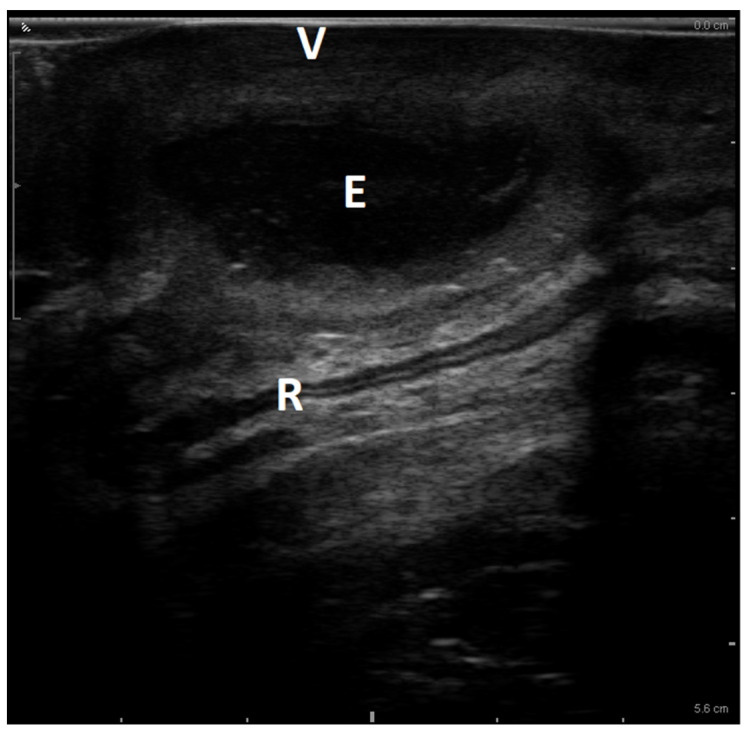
Transvaginal midsagittal sonographic view of the infected rectovaginal endometriosis, using an endocavitary linear probe. Ultrasound evaluation showed an inhomogeneous iso-hypoechoic 3–4 cm lesion involving the rectovaginal septum, but the integrity of the rectal wall is demonstrated and no fistula tract is observed. V = Vagina; R = Rectum; E = superinfected endometriosis.
